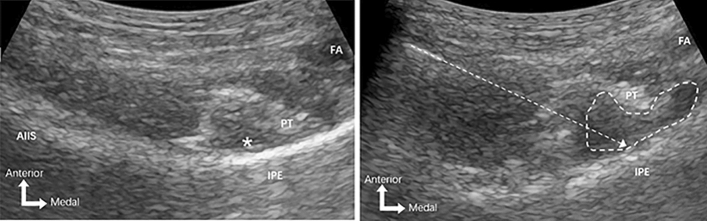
Images of ultrasound-guided pericapsular nerve group (PENG) block. The figure on the left shows the ultrasonic anatomy of PENG block; the needle tip was positioned between the psoas tendon and the pubic ramus using an in-plane approach. The figure on the right shows the local anesthetic spread following injection. Asterisk, target for local anesthetic injection; arrow, needle pathway; area circled by dashed line, local anesthetic spread; AIIS, anterior inferior iliac spine; IPE, iliopubic eminence; PT, psoas tendon; FA, femoral artery
